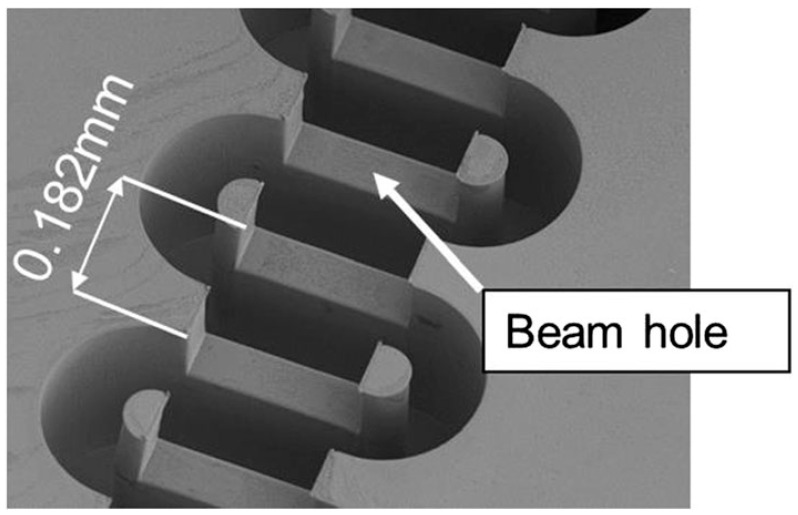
Trial 300 GHz FWG fabricated with X-ray LIGA [50].
